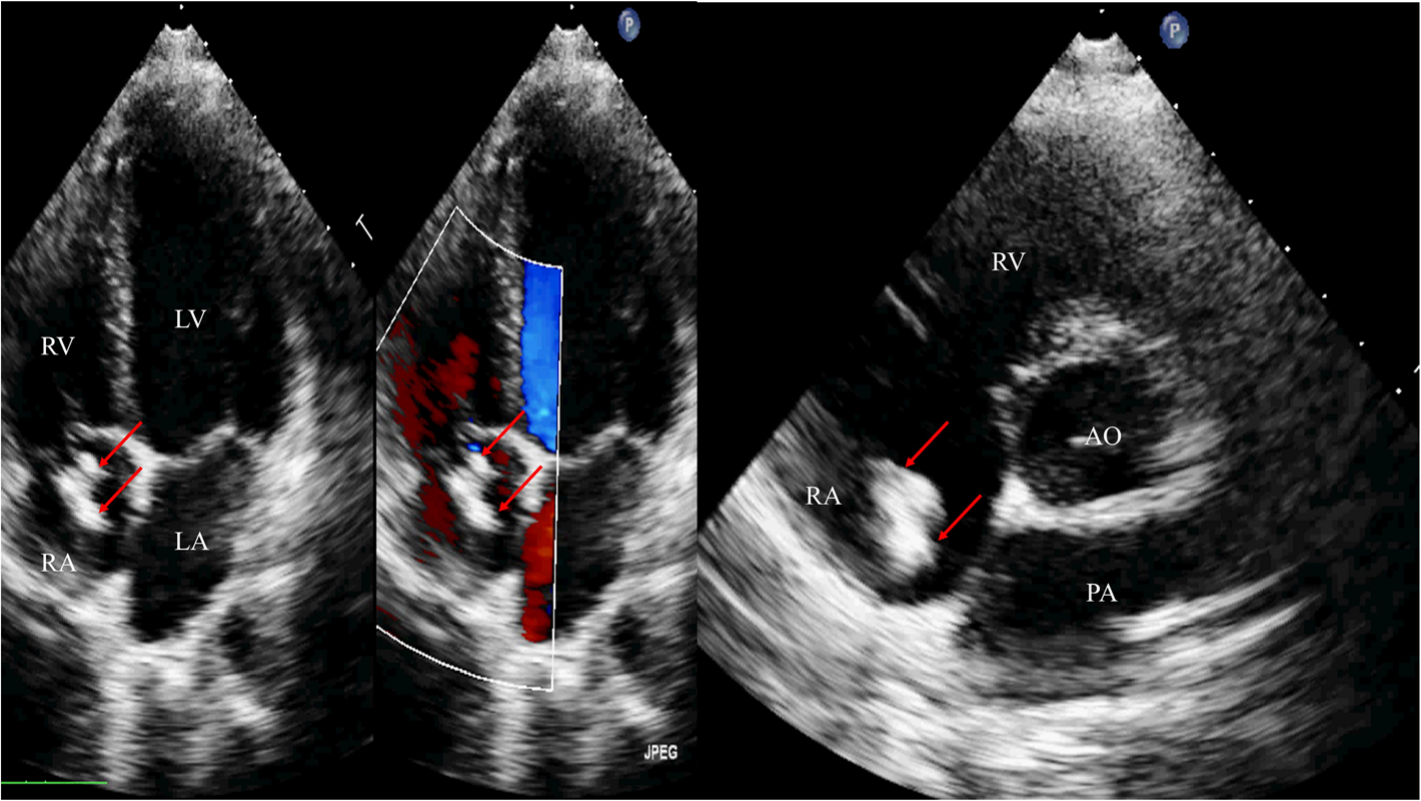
Echocardiographic imaging of the calcified thrombus. The apical four chamber view and the parasternal short axis view clearly demonstrate the large, mobile mass (arrow) originating from the free wall of the right atrium. RA—right atrium; LA—left atrium; RV—right ventricle; LV—left ventricle; Ao—aorta
